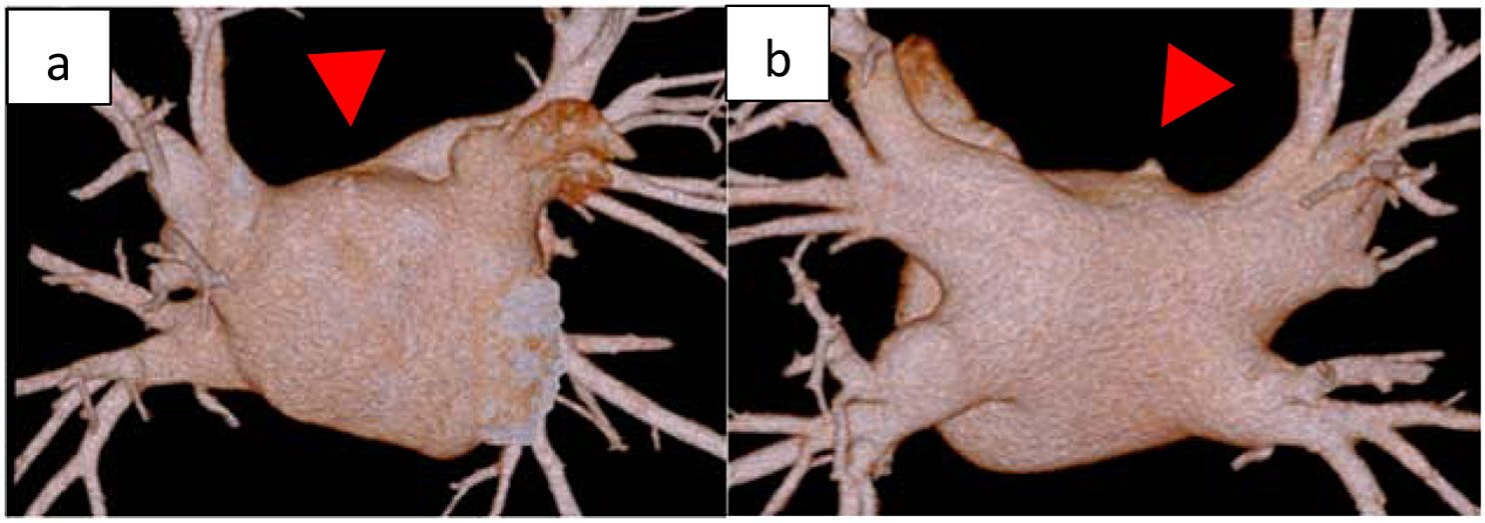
Case 1 a, b: Perioperative computed tomography showing a left atrial diverticulum (red arrow). a: Anterior left atrium, b: Posterior left atrium
